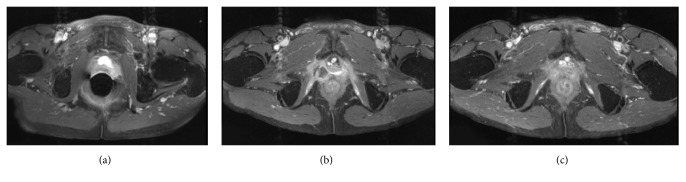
MRI revealing intact levator ani before HIFU (a), marked levator ani necrosis 2 months after HIFU (b), and regression of the levator ani necrosis after 3 years (c).
